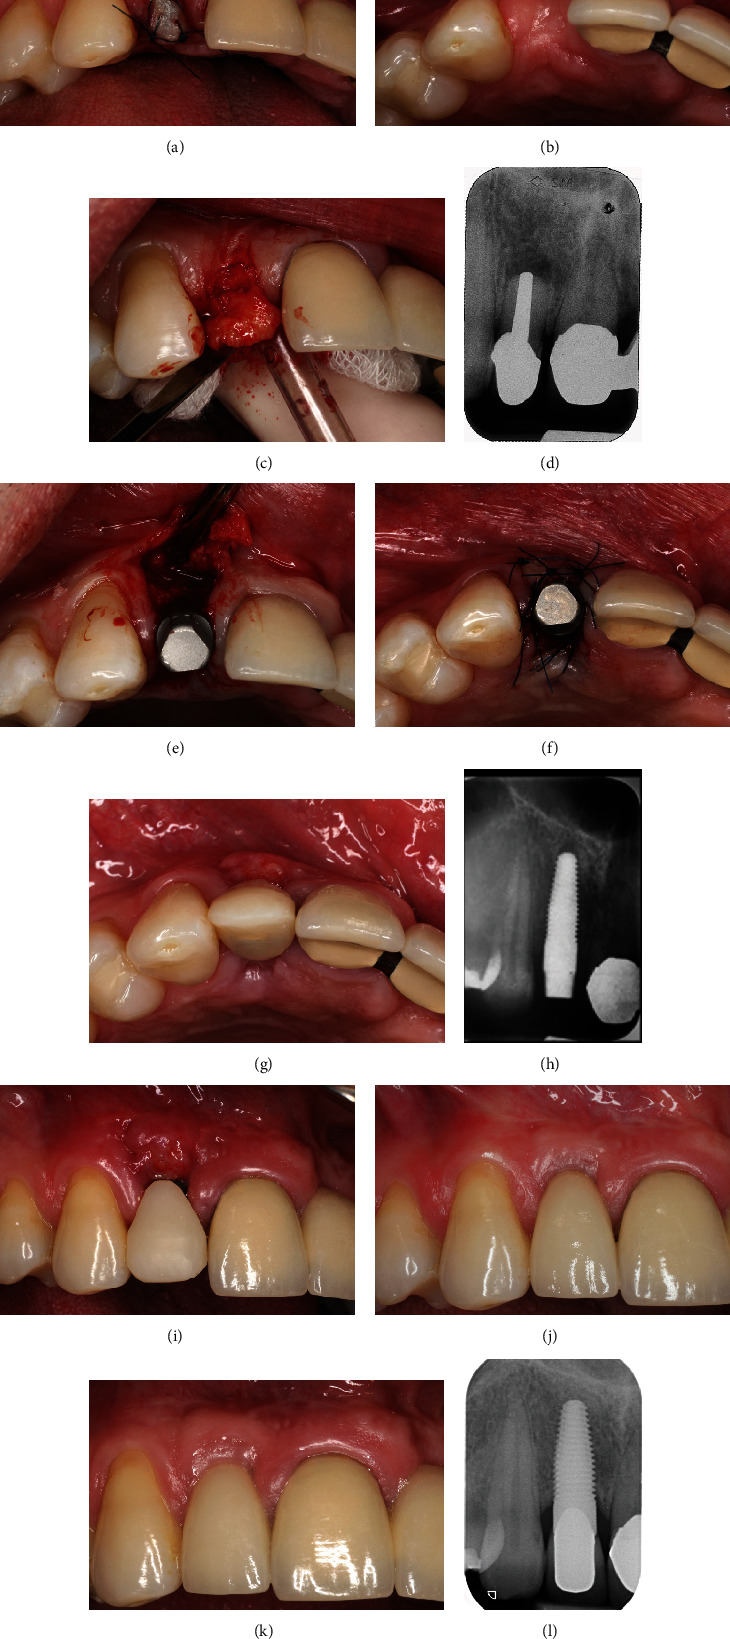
Delayed implantation of one-piece implant in esthetic zone: (a) clinical situation after extraction and socket preservation with soft-tissue augmentation; (b) clinical situation presurgically presenting small horizontal dimension of alveolar ridge; (c) reflection of connective tissue pedicle using roll technique with implant placement; (d) initial radiographic situation with hopeless tooth; (e) clinical situation after implant placement; (f) suturing and fixation of connective tissue at the buccal site; (g) healing after one week and suture removal, immediate provisional restoration (occlusal view); (h) radiographic control after implantation; (i) healing period one week after implantation with provisional restoration (buccal view); (j) prosthetic restoration with single crown four months after healing; (k) clinical situation 10 years after intervention; (l) radiographic control 10 years after implant treatment.
